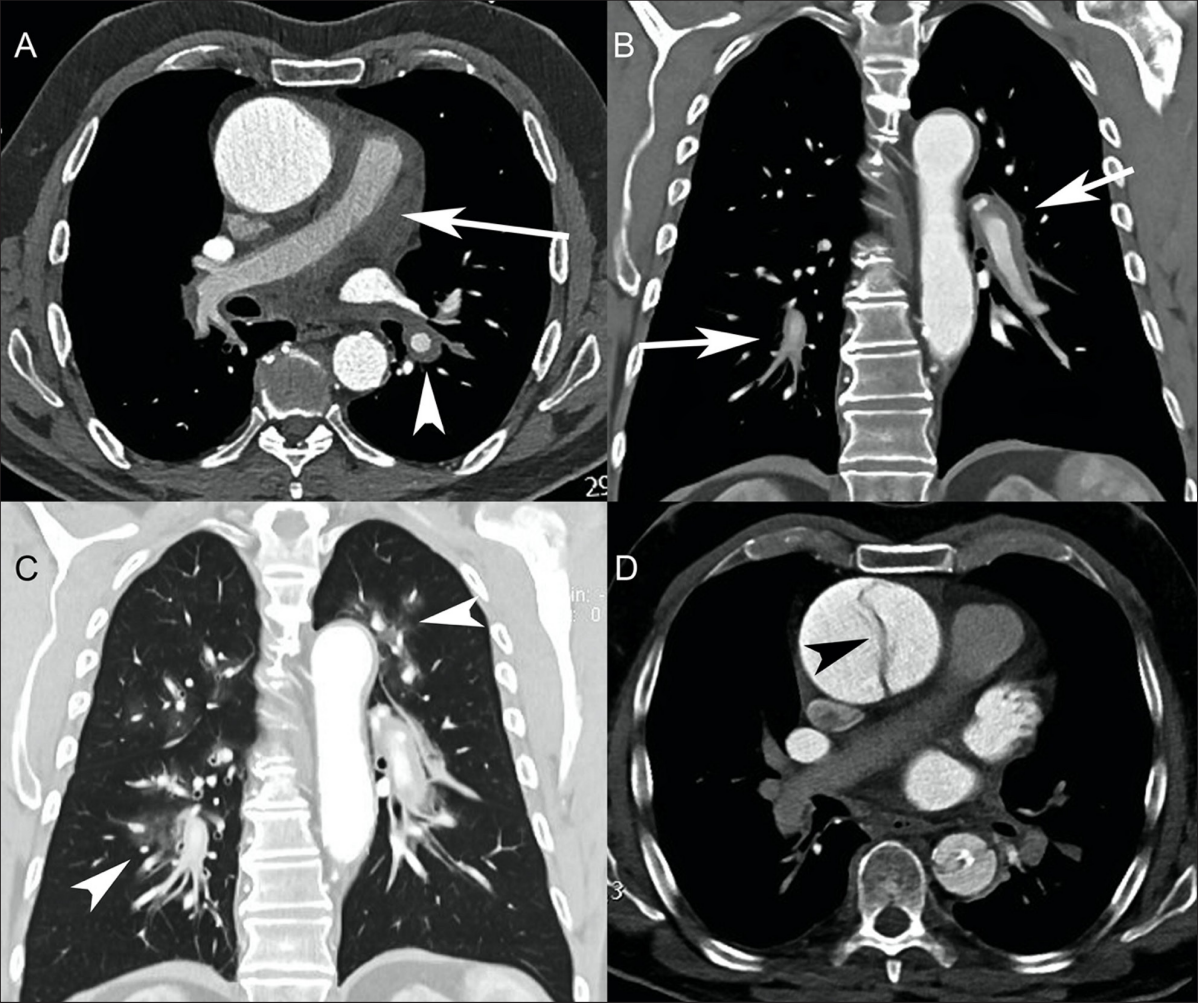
Patient 4. (A) Initial axial CT angiography (CTA) showed an aortic aneurysm. No intimal flap was seen. However, the contour of the ascending aorta was irregular. There was circumferential thickening of the pulmonary arterial wall (arrow), extending into the right and left pulmonary arteries (arrowhead), suggestive of pulmonary artery intramural hematoma. (B) Initial CTA (coronal reformation) showed the extension of the pulmonary artery intramural hematoma to the interlobar and segmental pulmonary arteries (arrows). (C) Initial CTA (coronal reformation, pulmonary window) revealed ground-glass opacities around the pulmonary arteries consistent with alveolar hemorrhage (arrowheads). (D) Second axial CTA performed two days later showed an intimal flap of the ascending aorta, suggestive of Stanford type A acute aortic dissection (arrowhead). Both the pulmonary artery intramural hematoma and alveolar hemorrhage had resolved.
